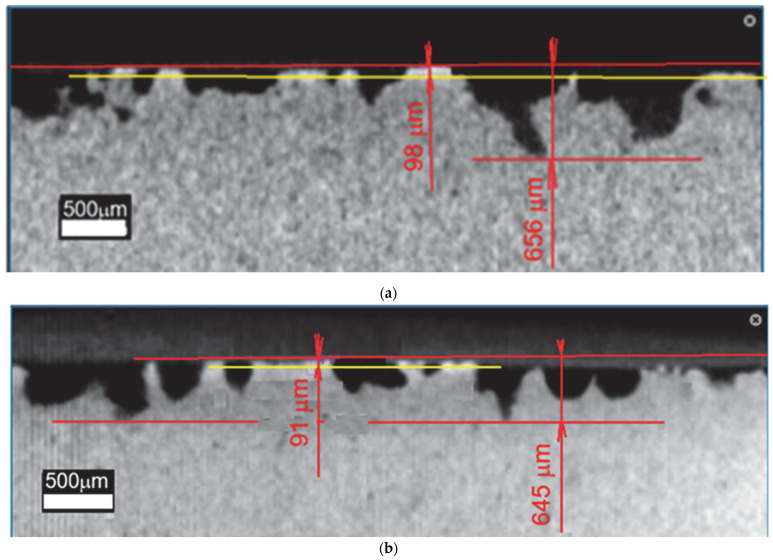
The CT images of the cavernous sinus depth of the: (a) 250/12 h state; (b) 250/24 h state.
